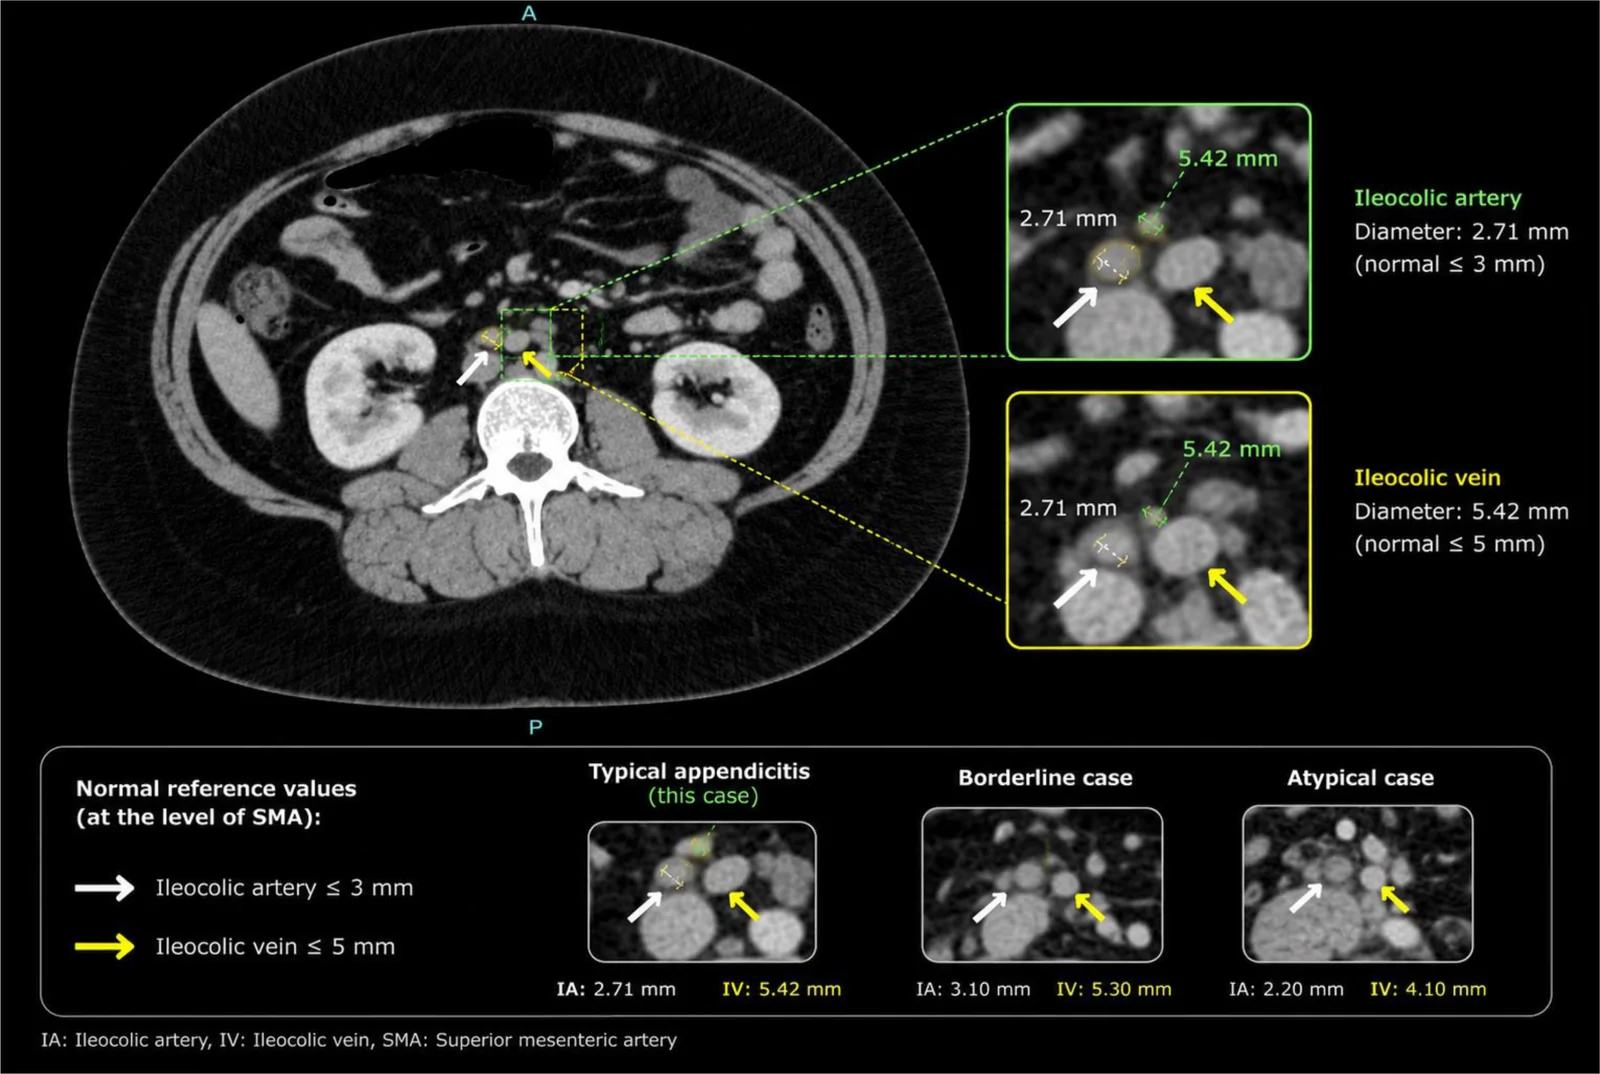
Axial contrast-enhanced abdominal CT image at the level of the superior mesenteric vessels in a patient with acute appendicitis, measurement of the ileocolic artery (white arrow) and ileocolic vein (yellow arrow). CT = computed tomography.
